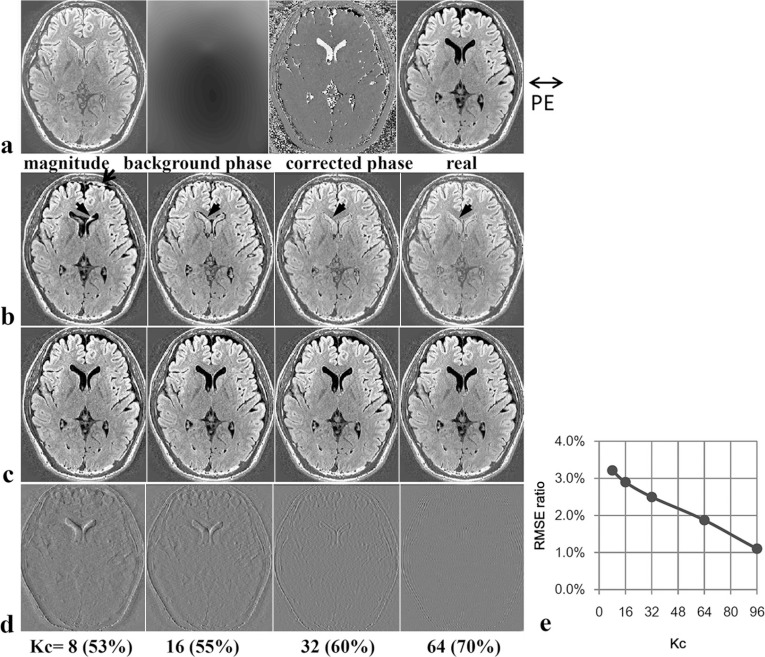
Results for T_2_-weighted (T_2_W)- fluid-attenuated inversion-recovery (FLAIR) brain data. (a) Fully sampled (−160 ≤ *k*_y_ ≤ 160) images (from left to right): magnitude image, estimated background phase image (with optimal low-pass filter for real-based polarity-preserving asymmetric Fourier imaging (RepAFI), distributed within *π* [rad] in the whole slice), corrected phase image, and corrected real image. (b) Standard AFI images obtained using partial data for several *K*_c_ values. (c) RepAFI images obtained using partial data for several *K*_c_ values. (d) Images obtained by subtracting the fully sampled corrected real image in the rightmost column of a from the corresponding RepAFI image in (c). (e) Root-mean-square-error (RMSE) ratio vs. *K*_c_ for (d). The errors (indicated by arrows) in standard AFI were corrected by RepAFI. In comparison with the fully sampled real image (top right), *K*_c_ ≥ 8 was necessary to preserve the basic structure in brain tissue, but *K*_c_ ≥ 32 was better for preserving detailed contrast.
